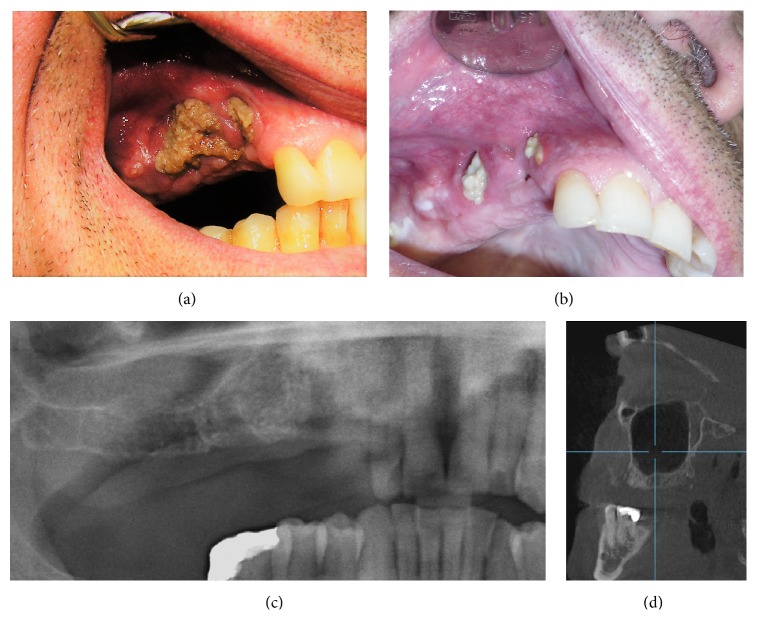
(a) Patient with medullary thyroid cancer, before and after conservative treatment. Patient just performed brushing exposed bone with stick or spatula with chlorhexidine 0,12% solution. Significant improvement (b). Panoramic radiography (c) and cone beam computer tomography rolled out bone sequestrum. (d) Scanora 3Dx device (*On Demand Software Cybermed, Seoul, Korea.)*
